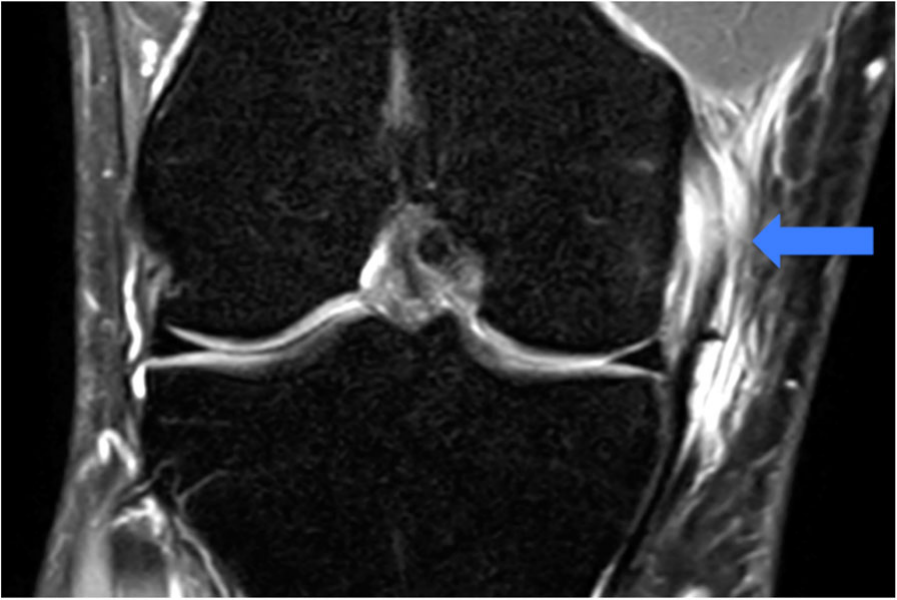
Knee Medial Collateral Ligament tear proximal, MRI T2 image with a proximal hypersignal of the MCL representing by the blue arrow
